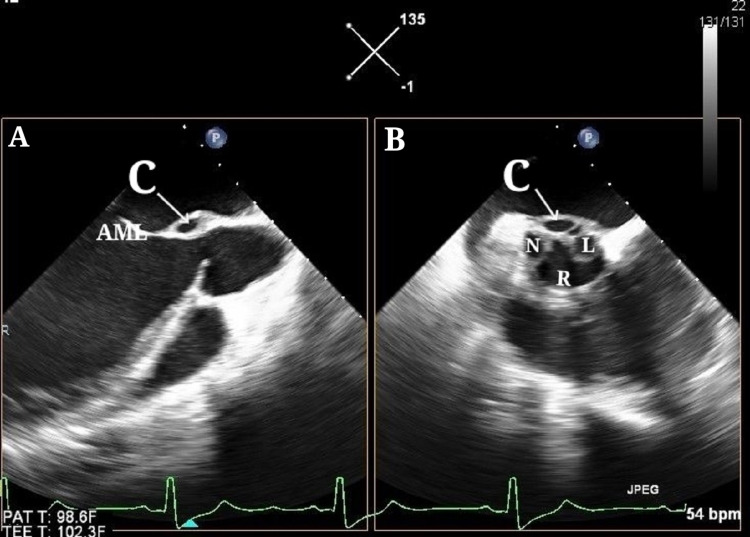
Transesophageal echocardiogram (A) Orthogonal image 90-degree showing the cavity near AML. (B) X-plane or aortic valve short axis showing the cavity at NCC-LCC commissure. C - cavity, N - noncoronary cusp, R - right coronary cusp, L - left coronary cusp, AML - anterior mitral leaflet.
